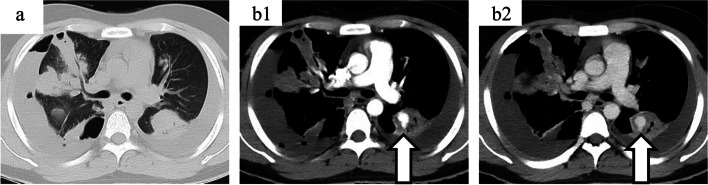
Chest contrast-enhanced computed tomography (CT) on day 8 of hospitalization. Figure 2-a shows a plain CT scan, while Figure 2-b1 and Figure 2-b2 depict the early and late contrast-enhanced phases, respectively. A newly developed right pneumothorax was observed (2-a), along with a pulmonary artery pseudoaneurysm in the left A6 (2-b1 & 2-b2)
